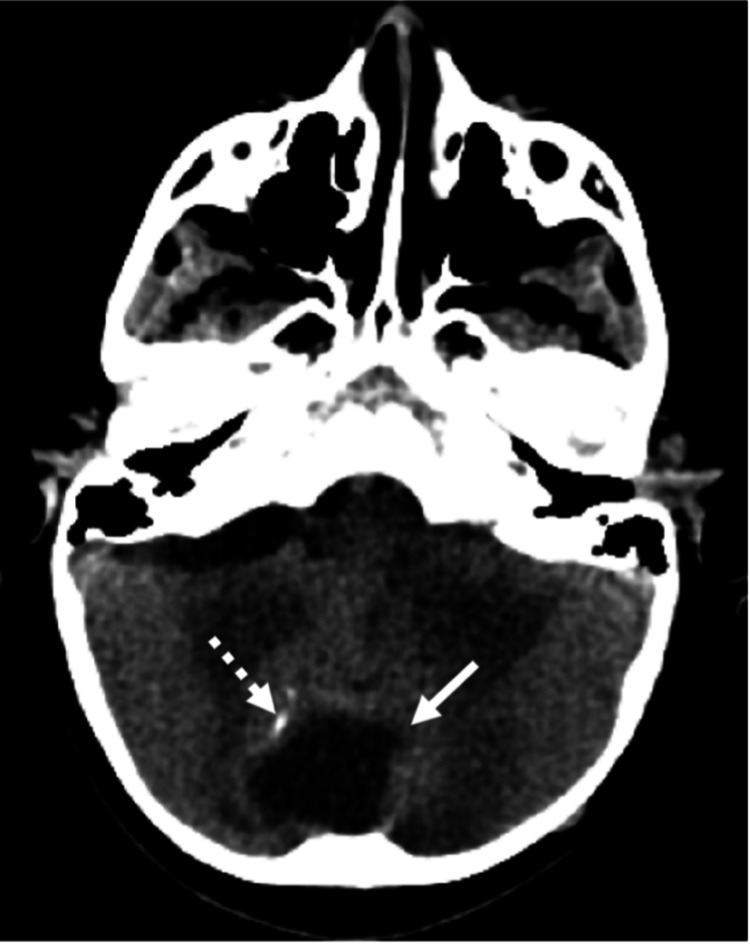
Axial CT (soft tissue window) showing a midline posterior fossa cystic mass (short arrow) with focal peripheral linear calcification (dashed arrow). CT, computed tomography
